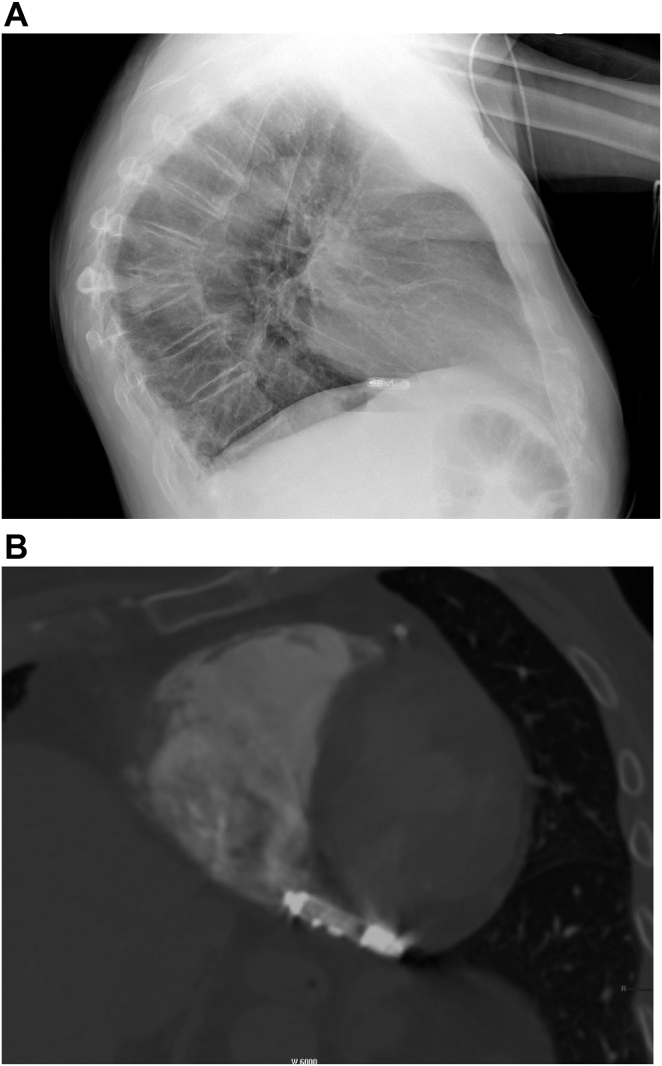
A: Lateral chest radiograph showing posterior dislodgment (in an uncertain position) of the Aveir (Abbott, Abbott Park, IL) leadless pacemaker (LPM). B: Computed tomography scan showing dislodgment of the Aveir LPM into the proximal part of the coronary sinus with the docking button protruding into the right atrium.
